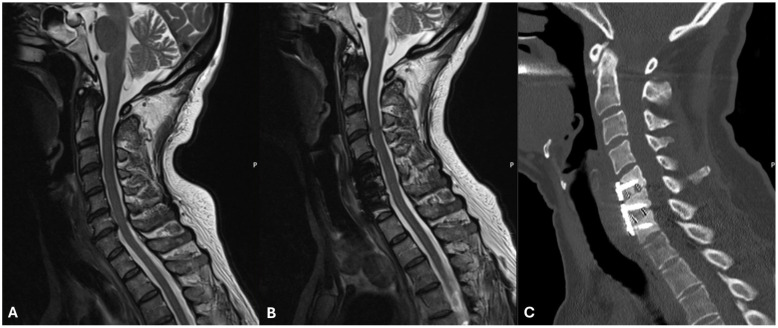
Preoperative MRI (A), as well as postoperative MRI (B) and CT (C) for case 1.
